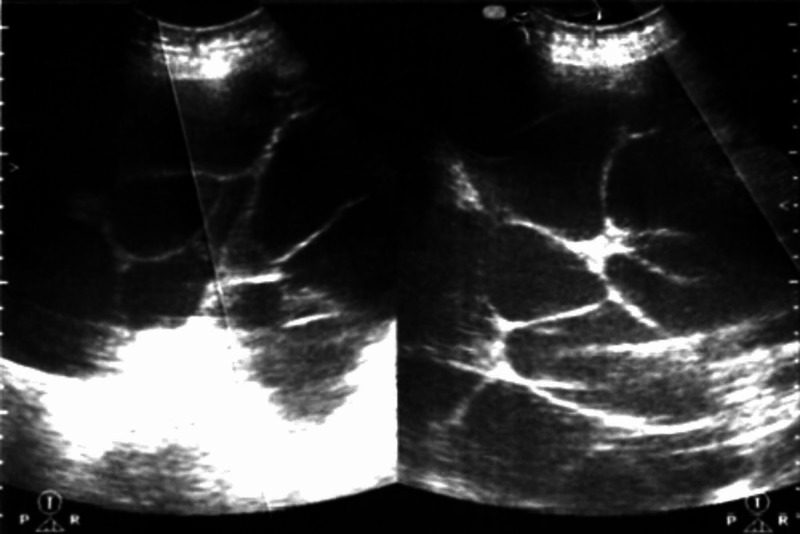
Ultrasound of ovaries showing bilateral cystic enlargement and a characteristic “spoke-wheel” pattern
